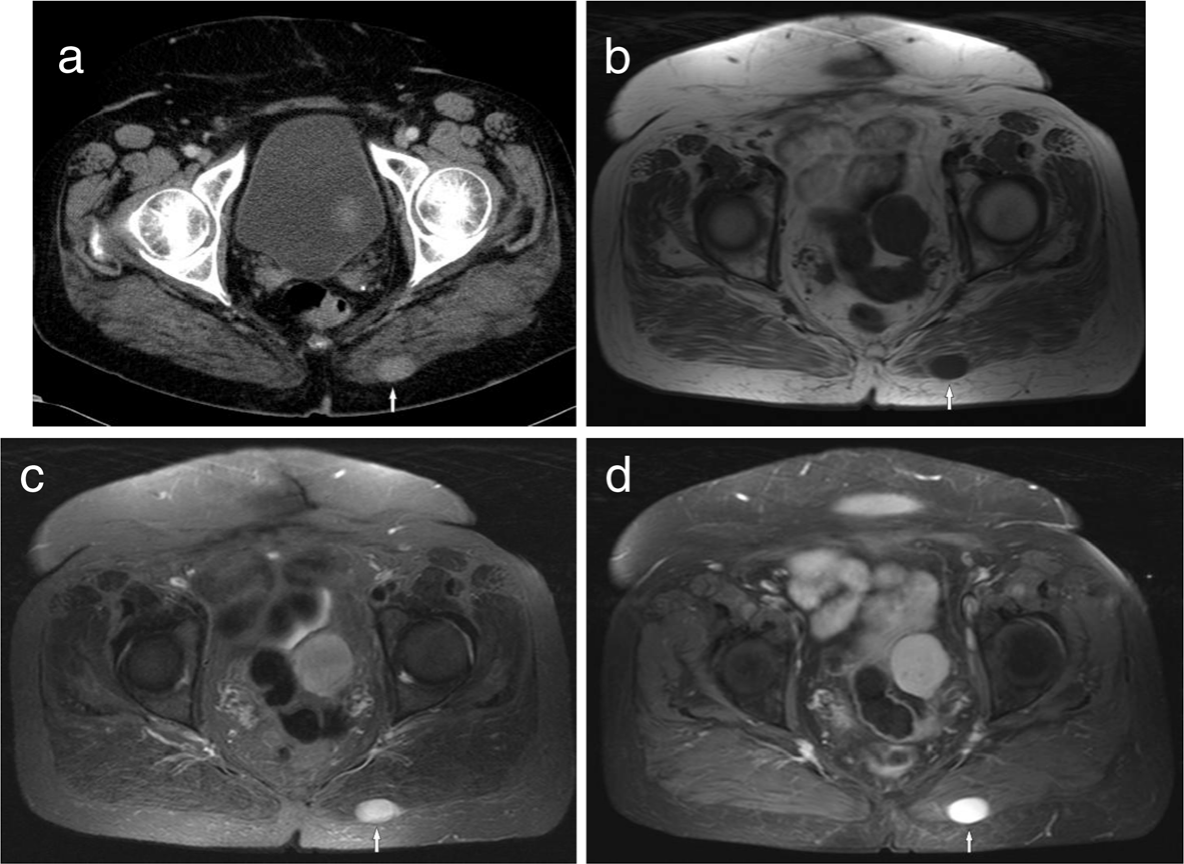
Imaging findings in a 50 year old woman with known history of melanoma. (a) CT image showing an oval mass with homogeneous contrast enhancement (type I) in the left gluteal musculature. (b) The lesion is hypointense on T1w image (spin echo pulse sequence, TR/TE: 569/11 ms). (c) The lesion is hyperintense on T2w image (short tau inversion recovery (STIR), TR/TE: 5210/80 ms). (d) After intravenous administration of contrast medium the lesion shows marked homogeneous enhancement (T1w spin echo with fat saturation, TR/TE: 610/12 ms).
